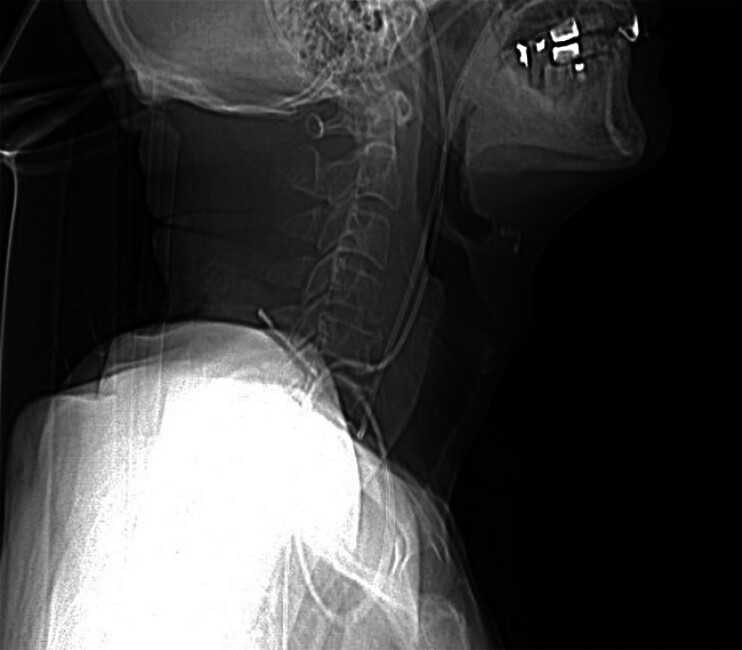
The follow-up CT scan revealing the drainage tube in an optimal position.
